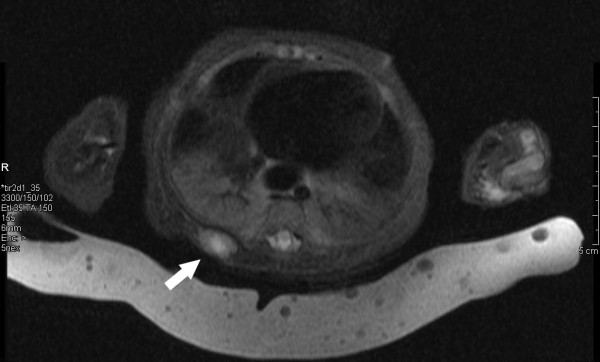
Magnetic resonance imaging of the right posterior hemithorax showing a soft tissue lesion. The nodule was inferior to the level of the scapula and was within the paraspinal muscles measuring 1.5 × 0.9 × 1.8 cm.
